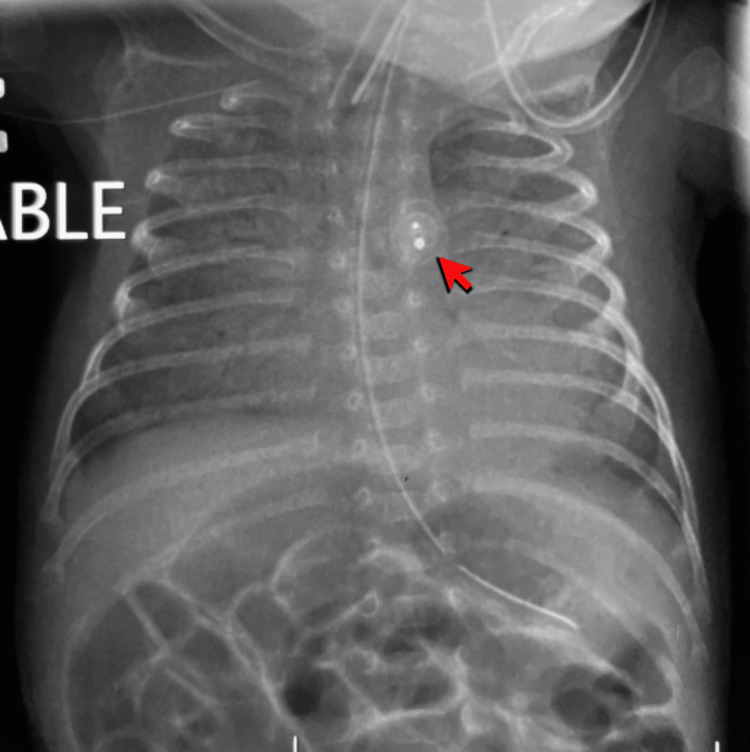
Following the procedure, the chest x-ray reveals a well-seated PDA device (indicated by the red arrow).
